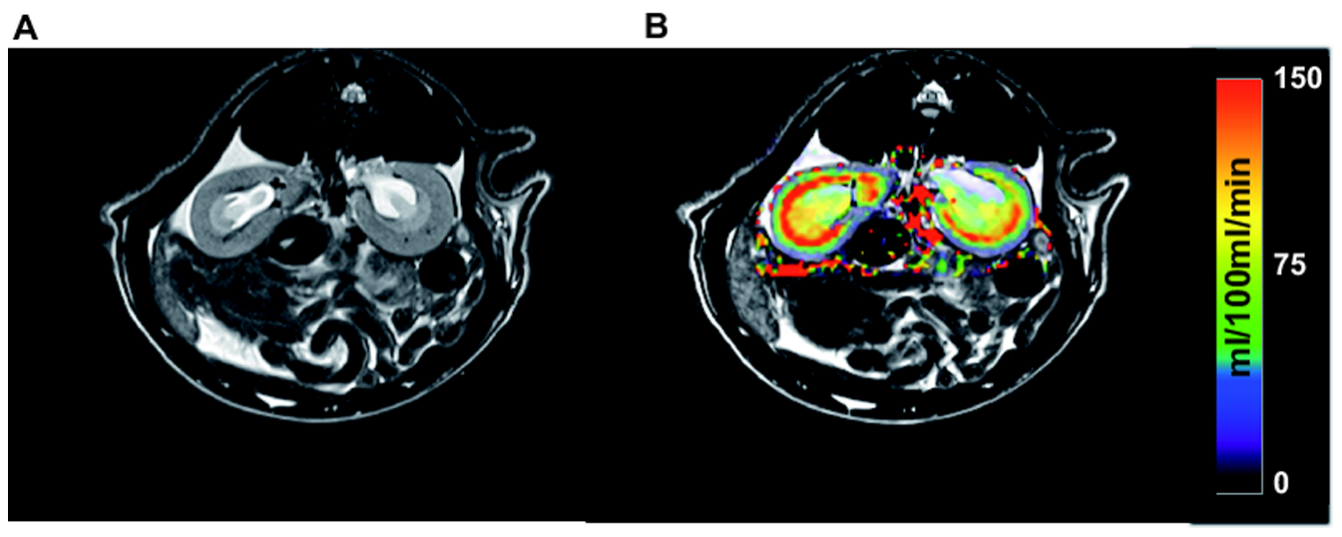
Image examples obtained by the MR measurements. A) depicts one slice of a T2 weighted morphological MR image used for cortex delineation, B) shows a parametric map of the tubular flow (filtration) calculated from the DCE- MRI perfusion data in units of ml/min/100 ml tissue. The map is superimposed onto the corresponding slice of the T2 weighted image.
